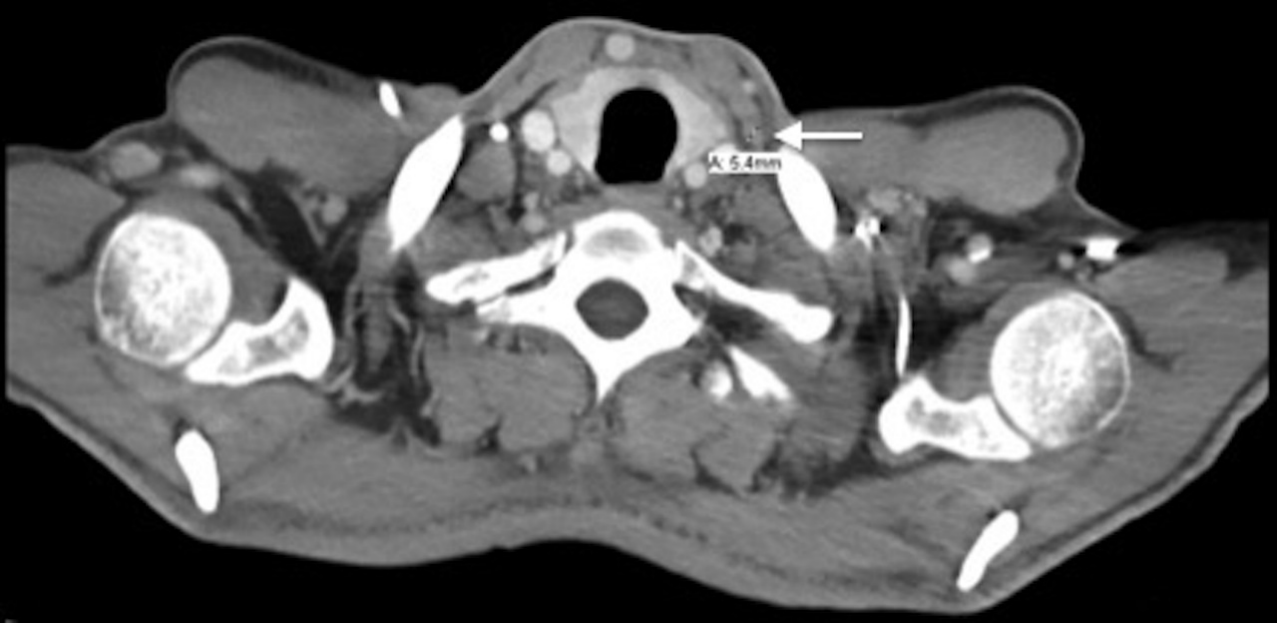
Transverse CT image taken from Patient 1 after four cycles of FOLFOX. This image shows that the left supraclavicular node decreased in size to 0.8 x 0.6 cm from 1.4 x 2.0 cm.
